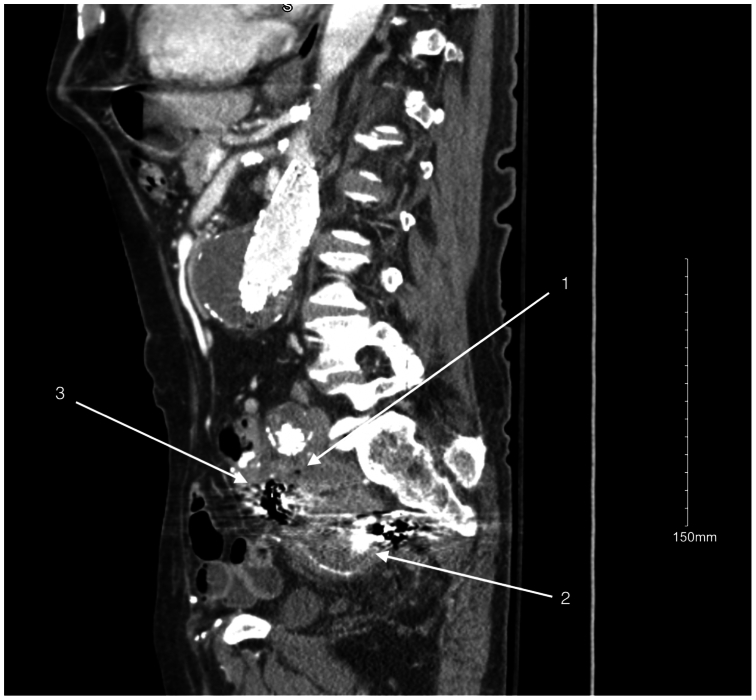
A preoperative sagittal computed tomography (CT) gas within the left hypogastric aneurysm sac (*1*), a patent left hypogastric artery (*2*), and coils that have migrated within the enlarging left hypogastric aneurysm sac (*3*).
